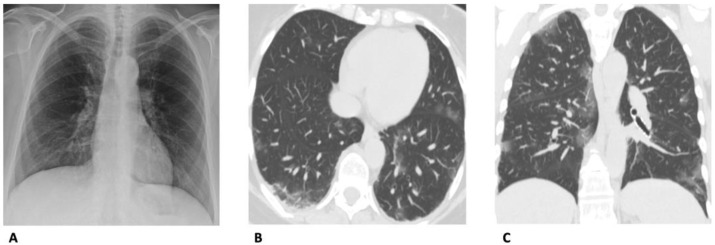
Comparison between CXR and CT findings in a 62-year-old woman with SARS-CoV-2-related pneumonia. (A) Anteroposterior chest X-ray was reported negative for lesions of SARS-CoV-2-pneumonia. The subsequent CT in the axial (B) and coronal (C) planes set with the parenchymal window width, however, showed multiple, bilateral, slight GGOs, especially in the subpleural areas.
